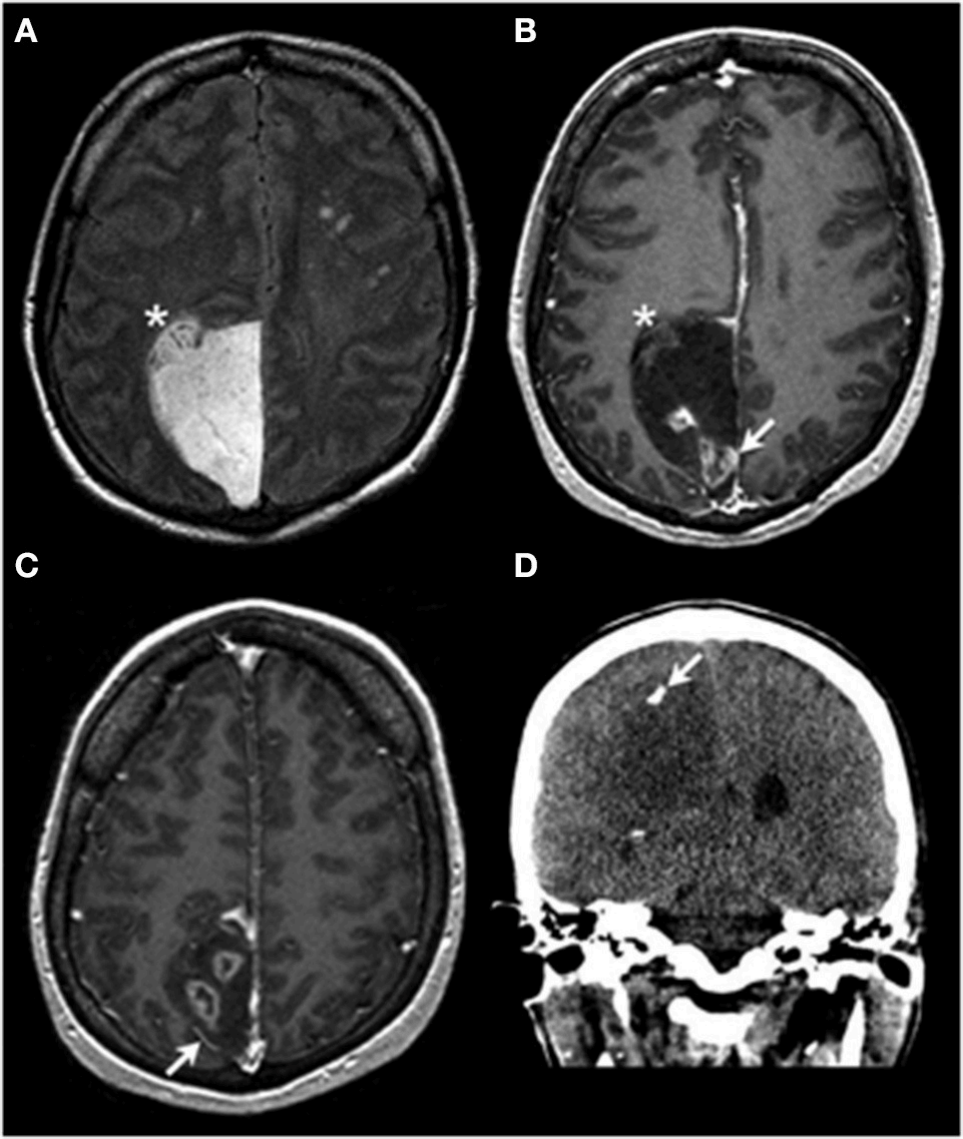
Radiologic findings. Axial T2-weighted FLAIR imaging (A) demonstrates an extra-axial hyperintense mass centered within the right paramedian parietal region with a focus that appears to be inseparable from the adjacent cortex (asterisk in A,B). On the axial T1 post contrast images (B,C) the lesion is predominantly nonenhancing and hypointense, with areas of heterogeneous enhancement (arrow in B). Also noted is lateral displacement of a cortical vessel (arrow in C), suggestive of an extra-axial location of the mass. Coronal non-contrast CT imaging demonstrates a focus of coarse calcification (arrow in D) in the hypoattenuating mass.
